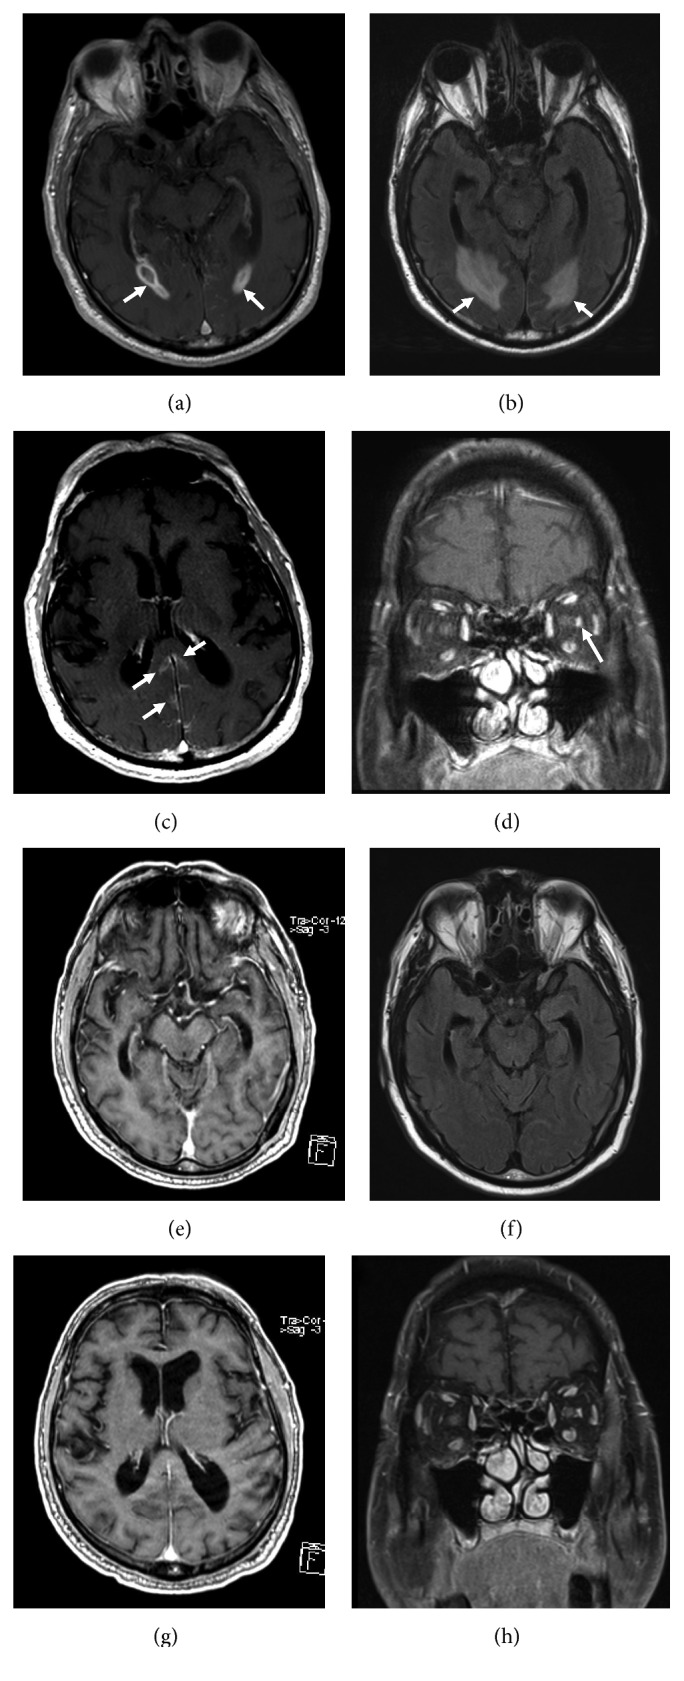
Magnetic resonance imaging of patient's brain obtained before and after initiation of ibrutinib. (a) Subependymal enhancement in December 2014 (T1 postcontrast). (b) Periventricular hyperintensity in December 2014 (FLAIR). (c) Leptomeningeal enhancement in December 2014 (postcontrast). (d) Left optic sheath enhancement in January 2015 (postcontrast). (e) Subependymal enhancement resolved in March 2015 (T1 postcontrast). (f) Periventricular hyperintensity resolved in March 2015 (FLAIR). (g) Leptomeningeal enhancement resolved in March 2015 (postcontrast). (h) Left optic sheath enhancement decreased in March 2015 (postcontrast).
